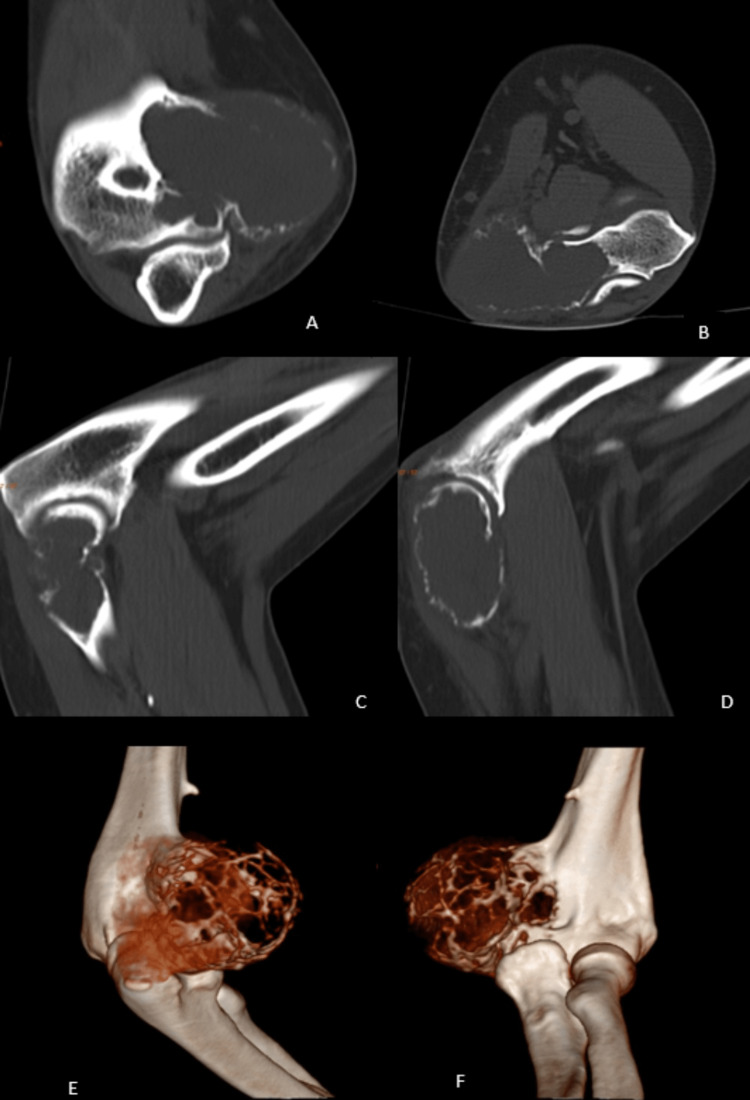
CT scans of the right elbow with 3D reconstruction (A) Coronal section of the right elbow swelling. (B) Axial section of the right elbow swelling. (C and D) Sagittal sections of right elbow swelling. (E and F) 3D reconstructed images of the bony swelling.
